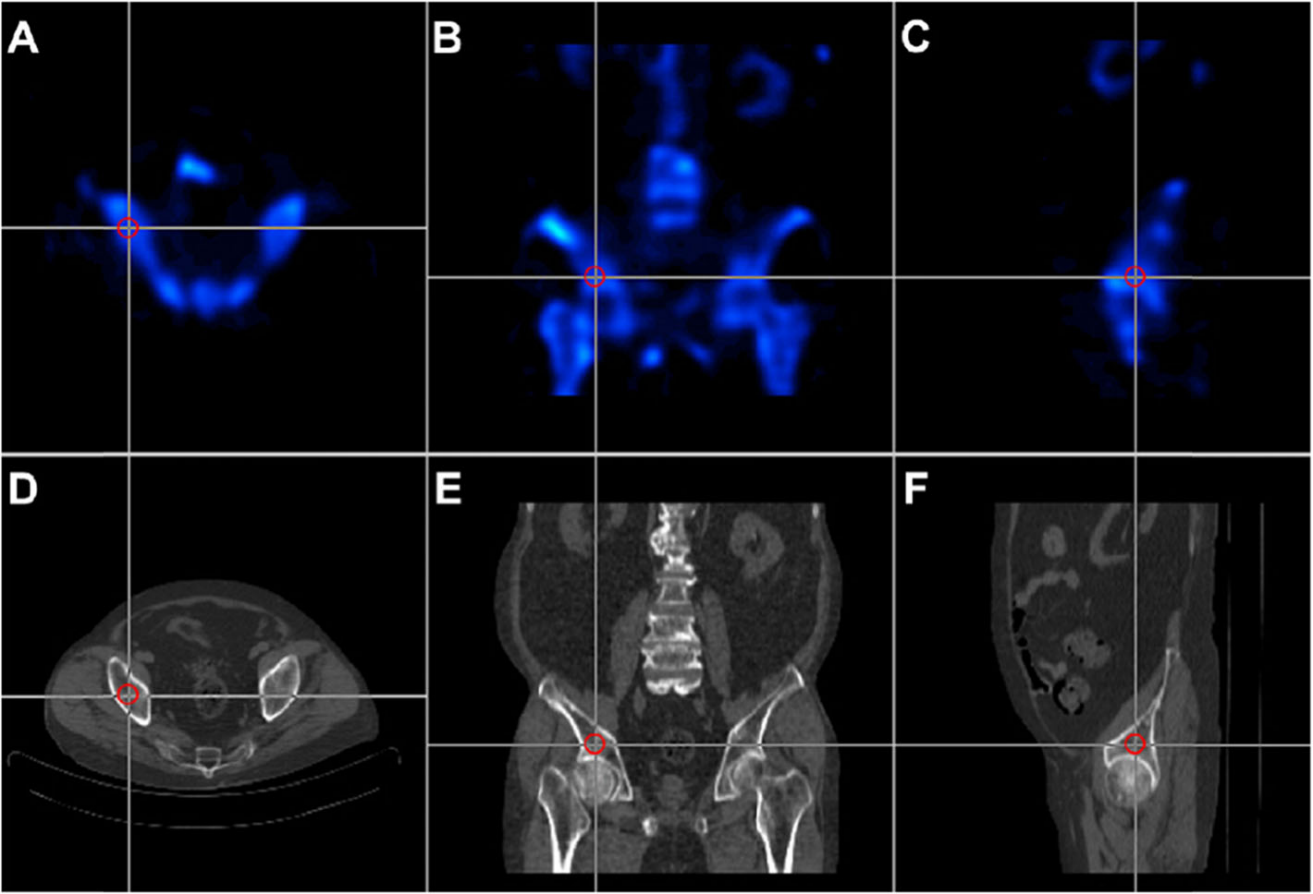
Quantitative analysis of the head-to-acetabulum uptake ratio with ROI in a 1-cm circle at the dome of the acetabulum in the affected hip. a,d ROI measured in axial view of SPECT/CT. b,e ROI in coronal view. c,f ROI in sagittal view. Three independently measured values of pixels are summated to an average value of ROI
